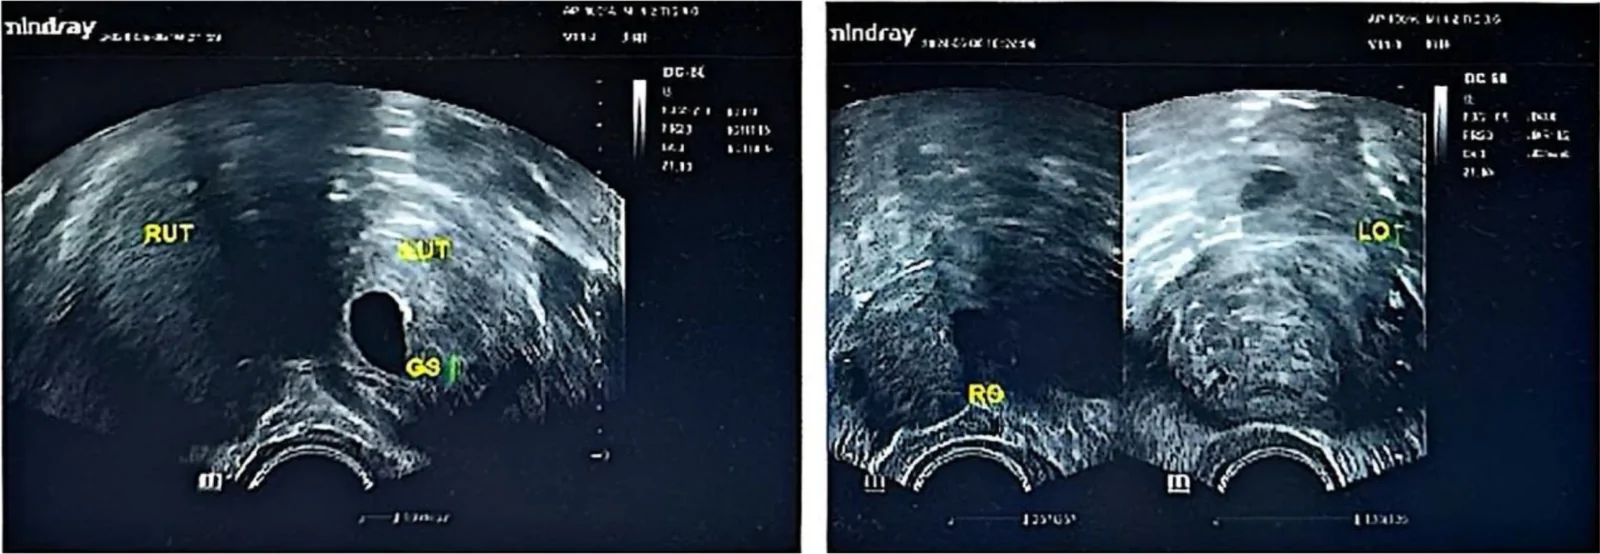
First-trimester transvaginal ultrasound confirming viable intrauterine gestation in the left cavity of a didelphic uterus.
